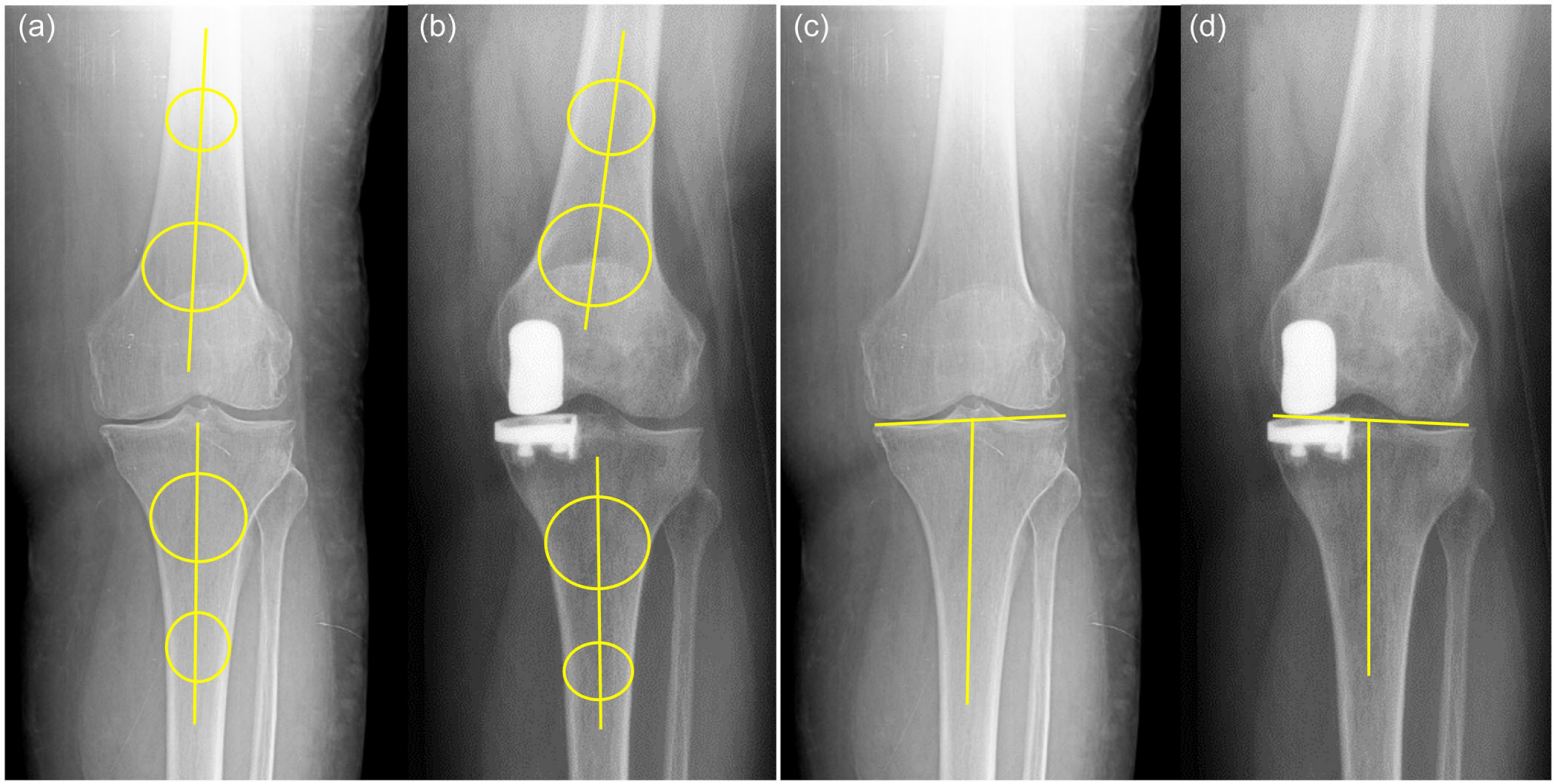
Measurement of knee joint alignment (a and b) and medial proximal tibial angle (c and d) on standing antero‐posterior direct knee radiographs.
